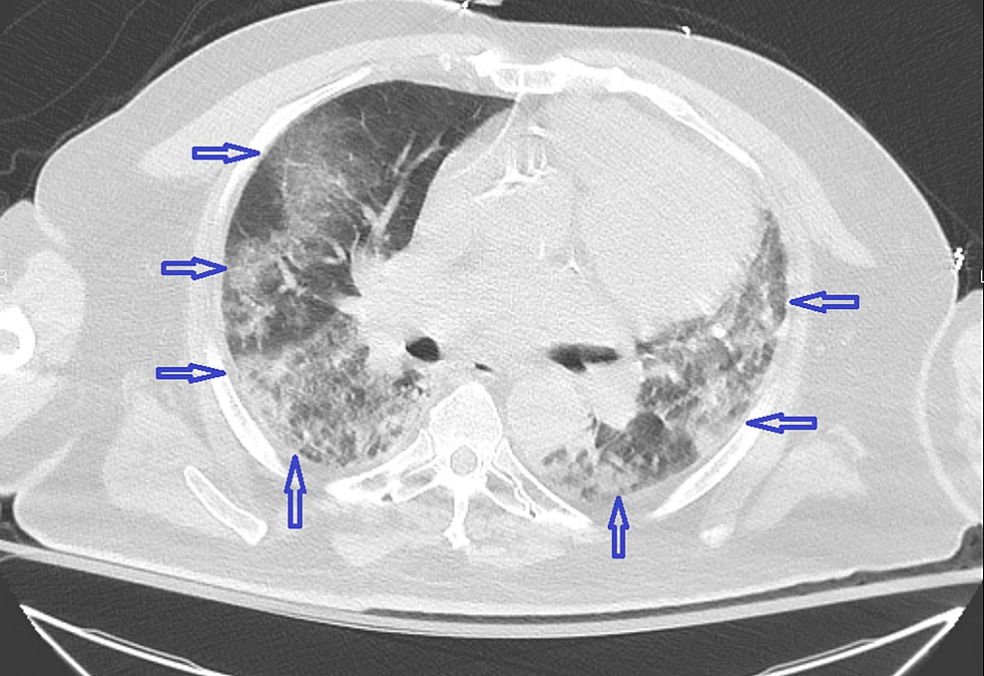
CT chest without contrast (cross-sectional view) demonstrating diffuse bilateral pulmonary opacities (blue arrows).
